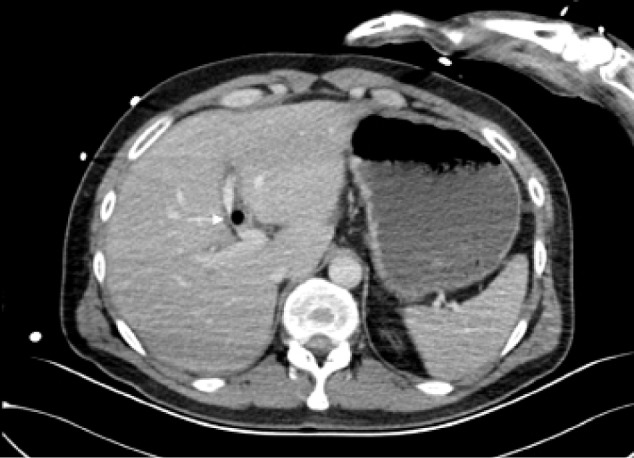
Computed tomography of abdomen showing the stick traversing the liver
